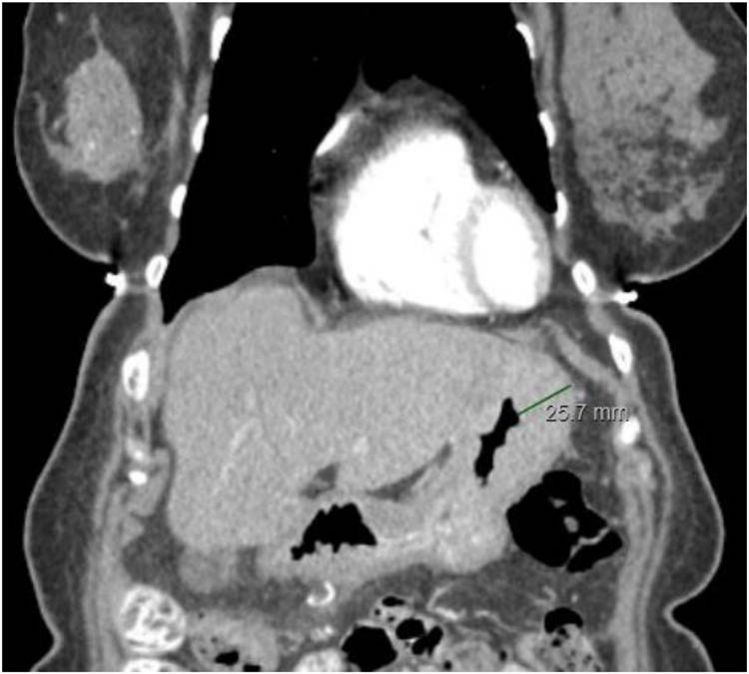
CT abdomen/pelvis displaying gastric wall thickening, coronal view.
